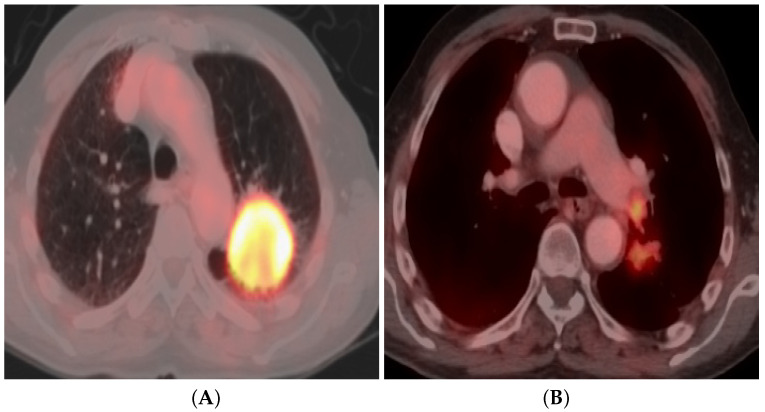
A 65-year-old male cigarette smoker with bronchogenic large-cell carcinoma. Axial-fused FDG PET/CT image (A) reveals FDG-avid large left upper lung lobe mass (5.5×6 cm) with maximum standardized uptake value (SUVmax) of 11.8. Axial-fused FDG PET/CT image at distal level (B) reveals FDG-avid ipsilateral hilar LN with SUVmax 7. No FDG-avid distant metastatic deposits, imaging-based staging IIIA (T3 N1 M0).
